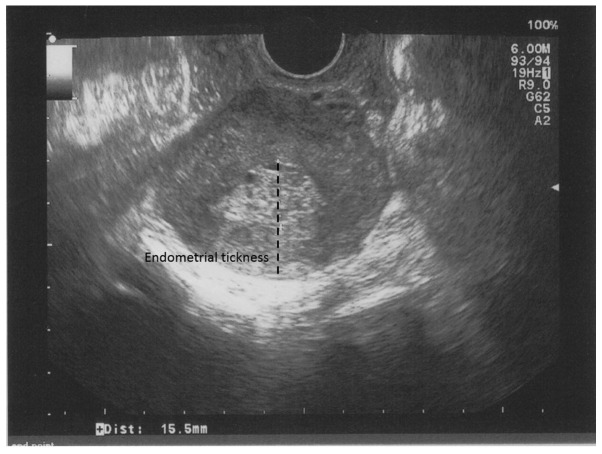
Case 1: Endometrial hyperplasia. Transvaginal ultrasonography at admission showed an endometrial hyperplasia with a hyperechogenic heterogeneous endometrial pattern with a thickness of 15.5 mm.
